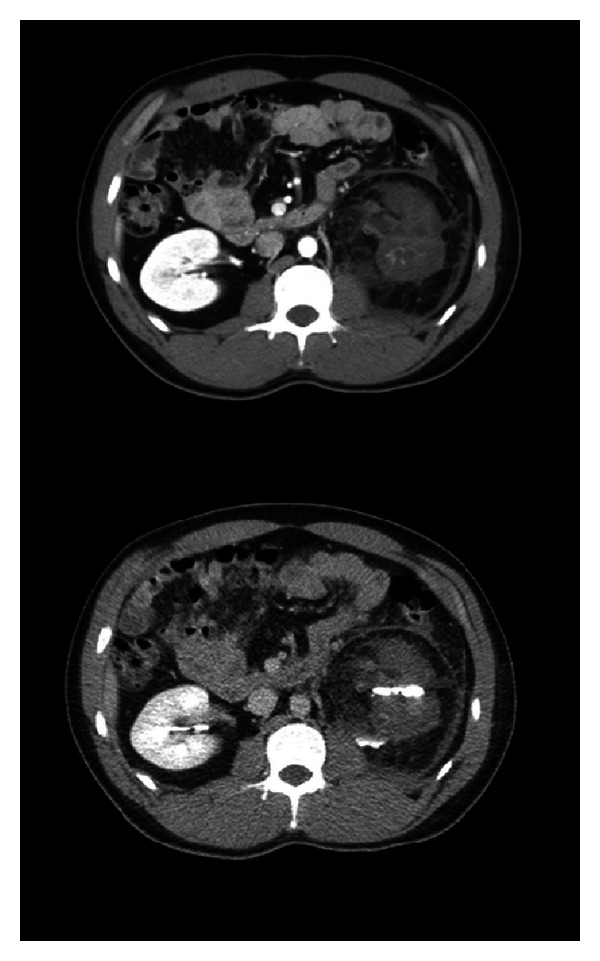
CT abdomen and pelvis with contrast upon presentation to ED showing Grade IV renal laceration.
